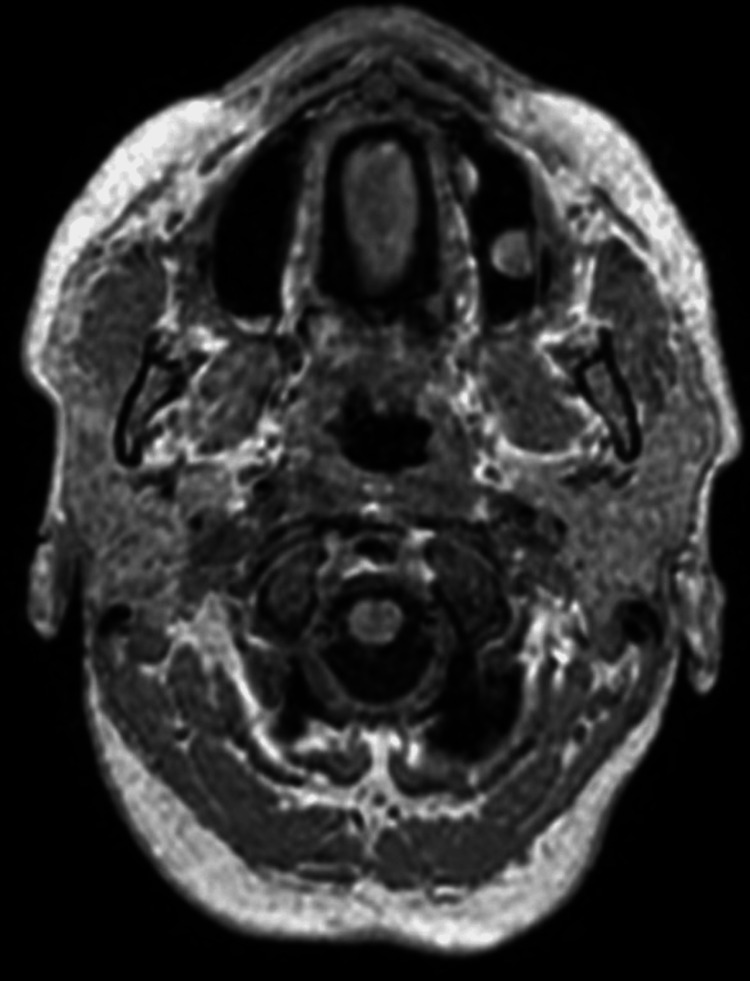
T1-weighted image depicts uniform signal, normal shape, no fat signal, and clear boundary with the surrounding tissue. Staging system for conventional imaging of the parotid glands proposed by Rao et al. [14], grade 0.
